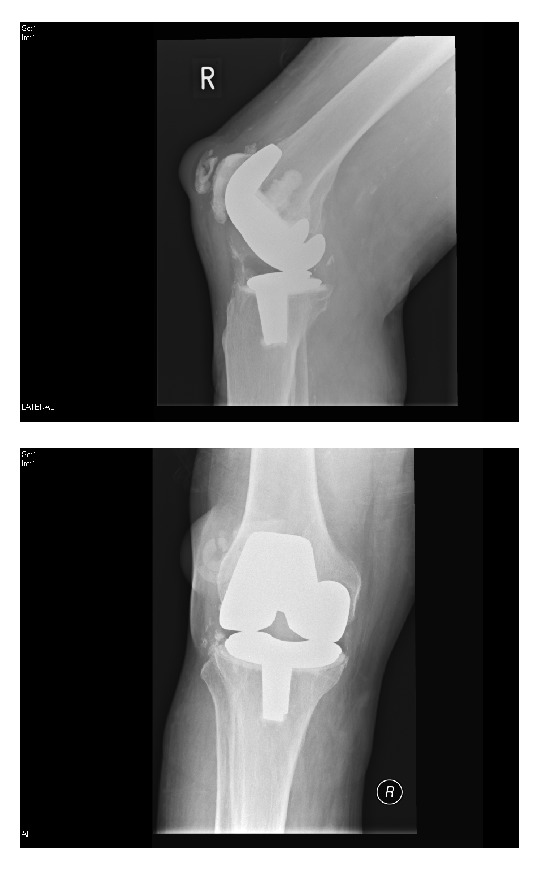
Postoperative AP and lateral radiographs of the right knee showing that the patellar button has migrated anteriorly to the patella.
